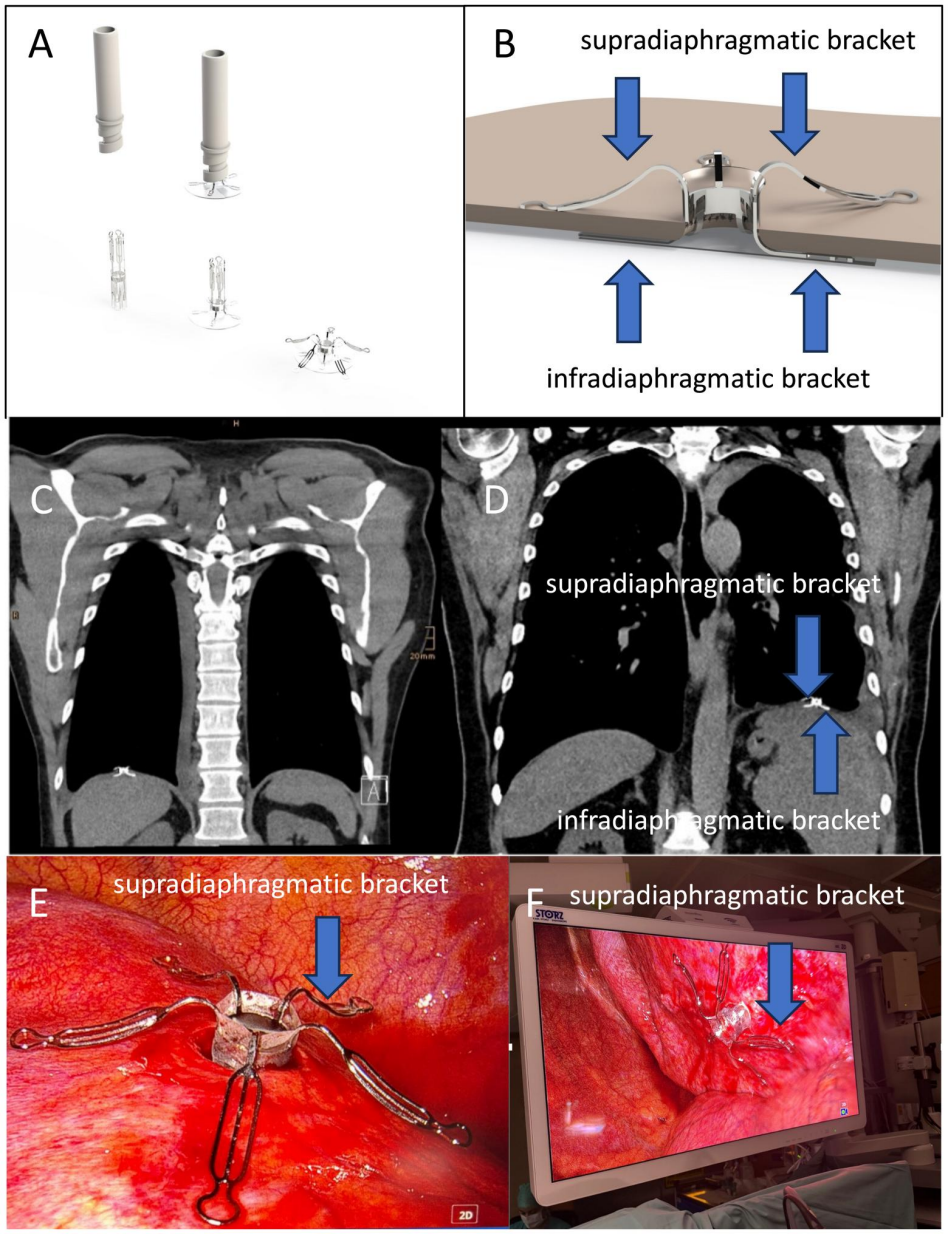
Deployment mechanism (A) and insertion situs of the diaphragmatic pleuro-peritoneal valve (B). The device has a diameter of 3 cm and consists of a thin infradiaphragmatic silicone sheet that integrates five one-way valves and a titanium scaffold with infra- and supradiaphragmatic brackets that retain the device in place and preclude obstruction of the pleural inflow by the lung. Frontal CT at follow-up (C and D) and intraoperative (E and F) views of the implanted valves in both patients. During implantation, the device is crimped into a custom-made delivery device, and the infra-and supradiaphragmatic portions are deployed individually.
